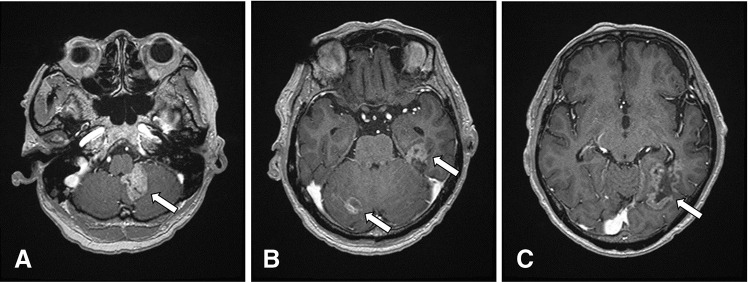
Head magnetic resonance imaging after neurological symptoms. Multiple enhancing lesions within the brain (arrows). (A) Cerebellum near left foramen of Luschka. (B) Hippocampus, right cerebellum. (C) Temporal-occipital lobe around the left lateral ventricle.
